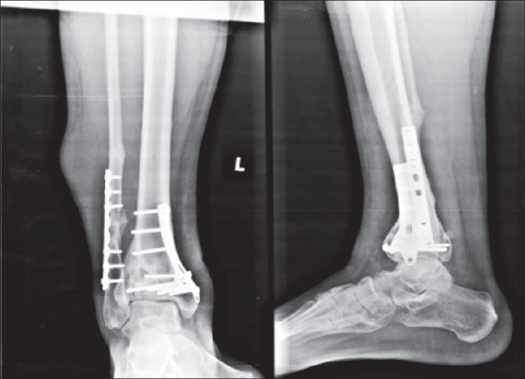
X-ray (AP and lateral view) of leg shows a soft tissue shadow on lateral aspect of leg
